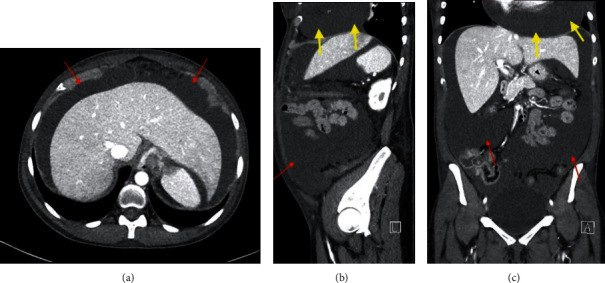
CT of the abdomen and pelvis with IV contrast on day 1. Mild bilateral basilar foci of atelectasis. Large pericardial effusion (yellow arrows in (b) and (c)). Large amounts of ascitic fluid (red arrows in (a)–(c)). Mild stranding of the peritoneum anteriorly.
